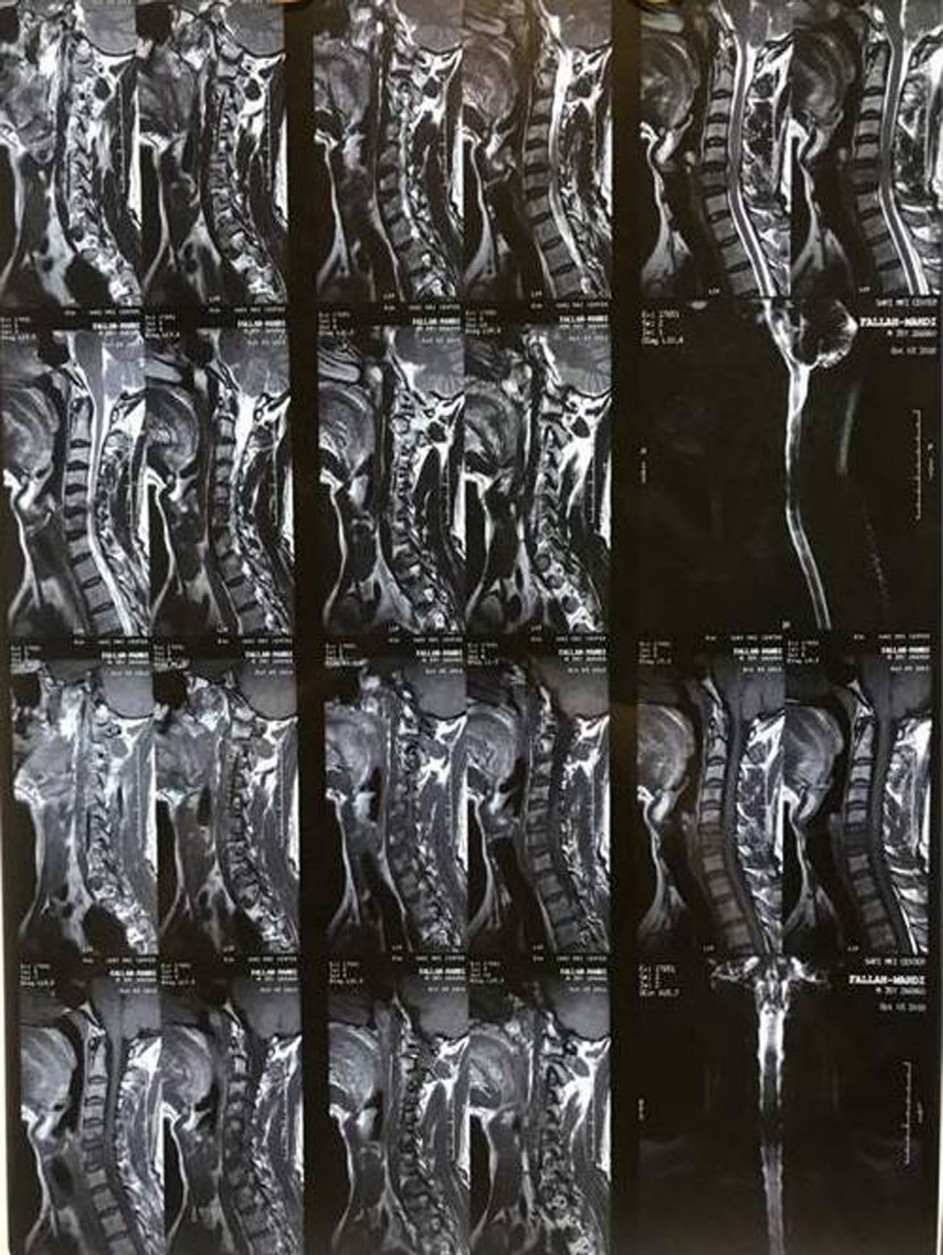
Cervical spine MRI shows an epidural abscess at the C5‐C6 levels
